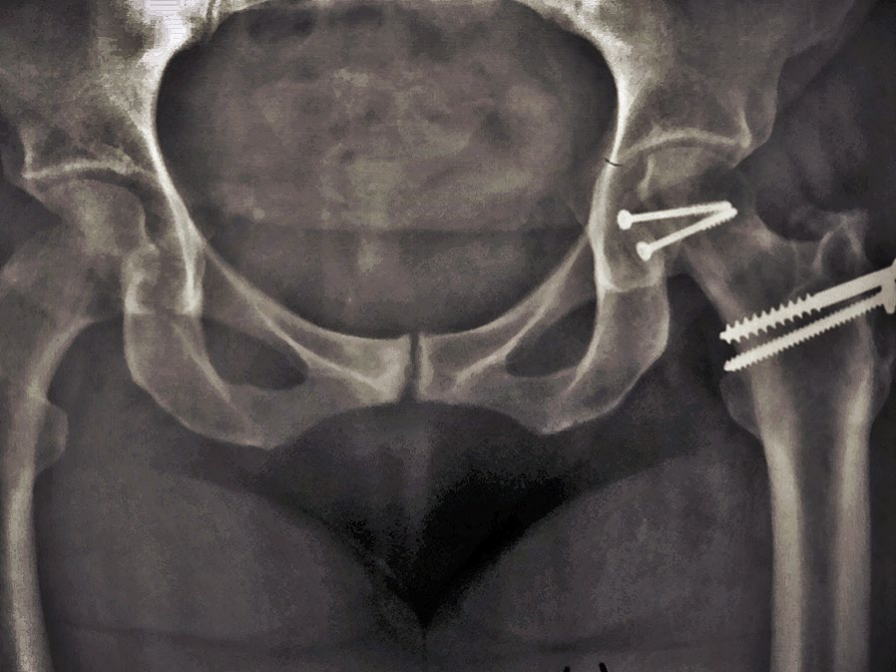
Follow-up radiograph 2 years postoperatively
